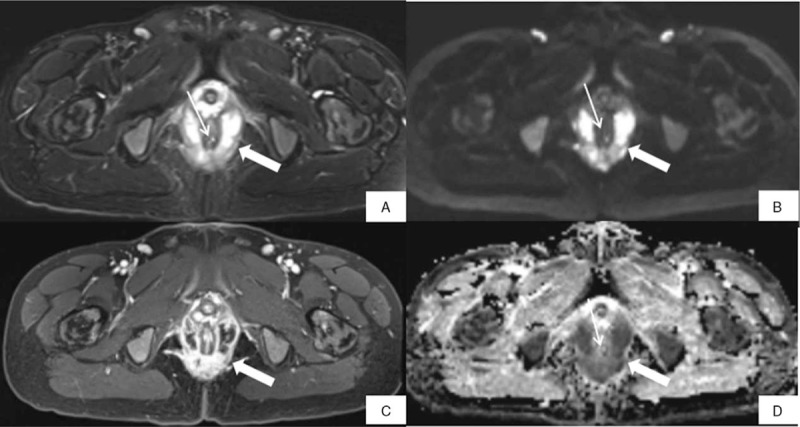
MR images of a 23-year-old man with a horseshoe fistula. (A) The internal orifice (thin arrow) is identified in the 5 o’clock direction, and the fistula tract (thick arrow) is noted around the anal canal on axial fat suppressed T2-weighted imaging (FS T2WI). (B, D) The internal orifice (thin arrow) and fistula tract (thick arrow) are both obviously showed on axial diffusion-weighted imaging (DWI) and the corresponding map of apparent diffusion coefficient (ADC). (C) The internal orifice is not identified on axial fat suppressed T1-weighted imaging with contrast enhanced (FS T1WI+CE), which maybe the result of the enhancement of the internal orifice and the mocosa at the same time. The fistula tract is visible (thick arrow).
